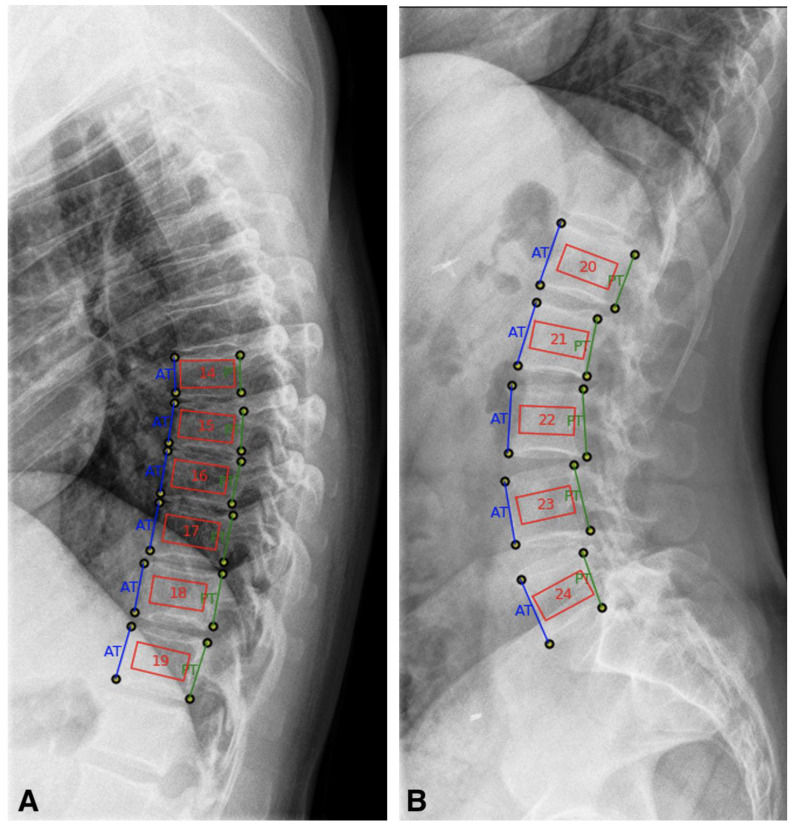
Application of TX-Analyzer™ software on lateral-view radiographs of the thoracic and lumbar spine of patients with Crohn’s disease and controls. Definition of region of interest (ROI) using a preset biopsy mask, after definition of the upper and lower and the anterior and posterior corner of the vertebral bodies the ROI box is placed by the software. (A) thoracic spine and (B) lumbar spine.
